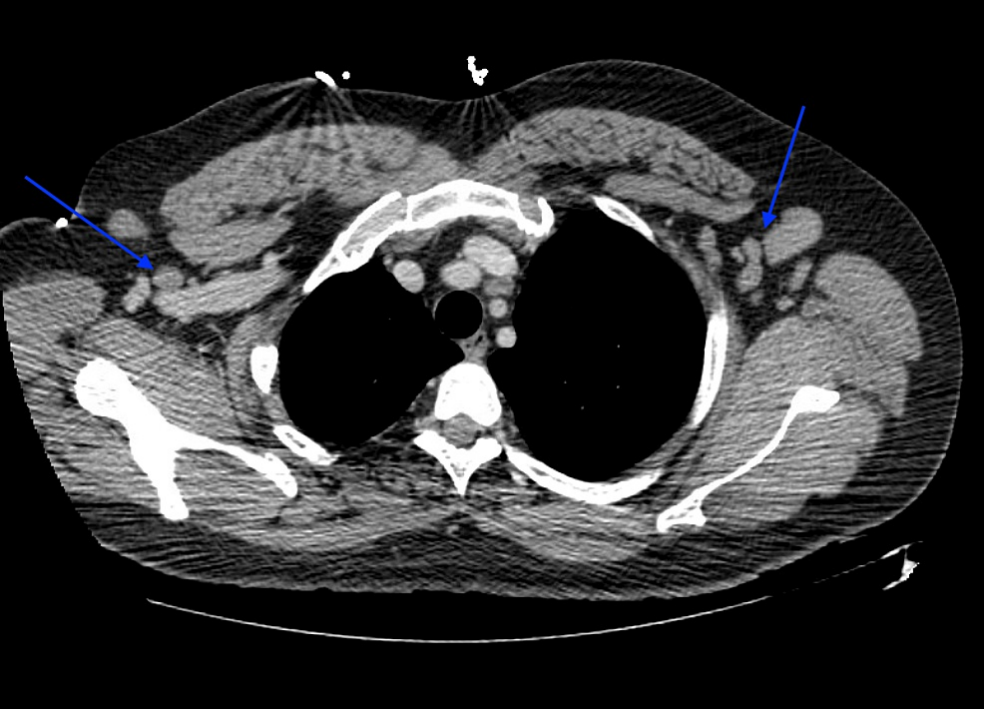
CT of the chest showing bilateral axillary lymphadenopathy (blue arrows)
